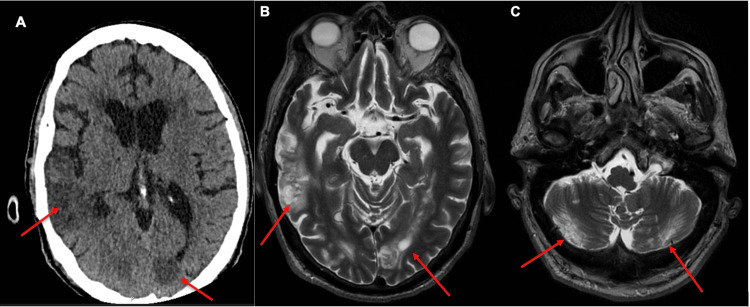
(A) Computed tomography showing bilateral infarcts involving both the anterior and posterior vascular territories (arrows); (B, C) T2 Periodically Rotated Overlapping Parallel Lines with Enhanced Reconstruction (PROPELLER) images showing bilateral cerebral and cerebellar infarcts (arrows), respectively.
